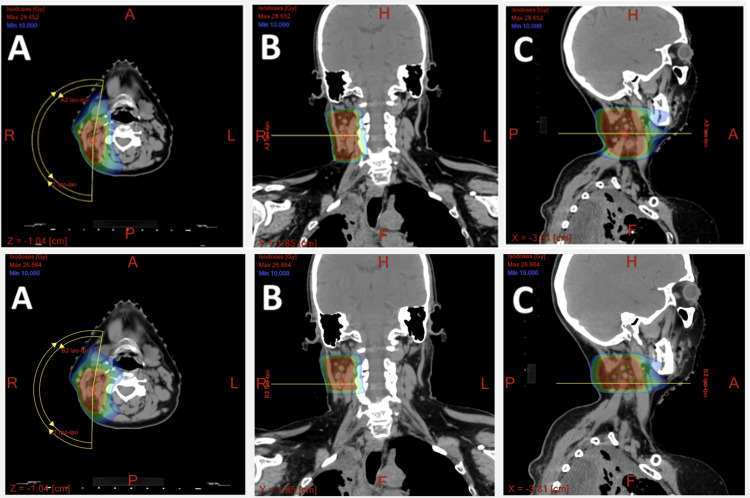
CT scans of the target radiation field with a color wash display of the dose (at a minimum of 10 Gy) for a total 50 Gy plan showing the dose distribution in the single-isocenter plan. Gross tumor volume is highlighted in red. Top row = right neck radiation. Bottom row = right neck boost radiation. A = transverse; B = frontal; C = sagittal.
